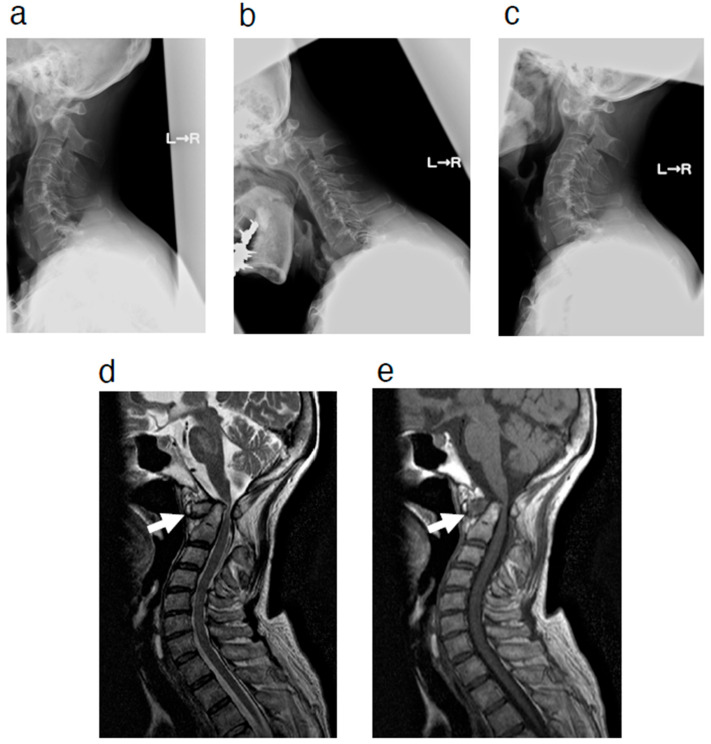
(a–c) Radiographic image at the follow-up visit. Lateral view in flexion (a), neutral (b), and extension (c) positions. (d,e) MRI at the follow-up visit showing an antero-odontoid pseudotumor (white arrows). Sagittal view of T2-weighted (d) and T1-weighted (e) images. MRI = magnetic resonance imaging.
